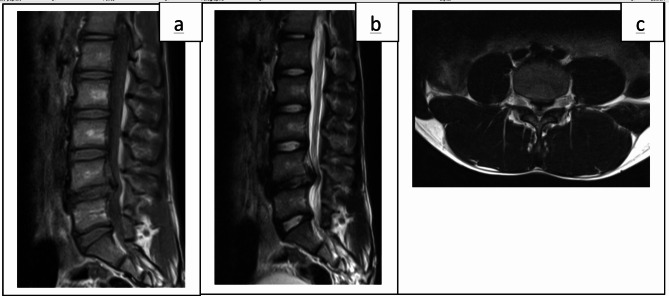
MRI images of the patient of the observation 3. Sagittal section in weighted sequence T1 (a); T2 (b); axial section in T2-weighted sequence (c) to highlight a compressive posteromedial L4L5 disc herniation; the disc L4L5 being always well hydrated
